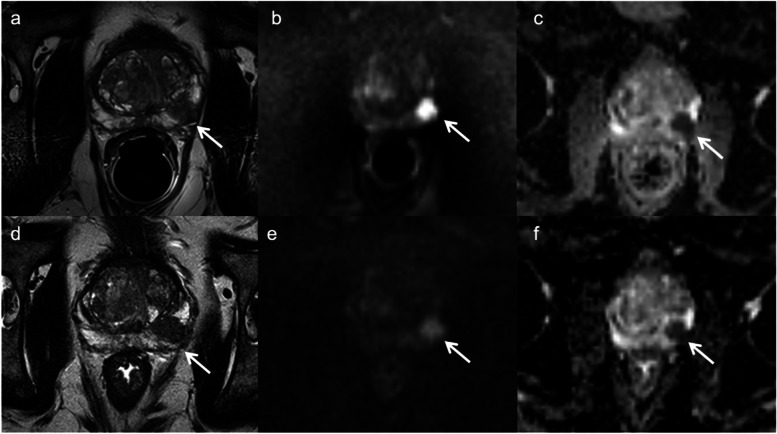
66-year old man (PSA of 9.03 ng/mL) who underwent MRI using a combined rigid two-channel phased-array endorectal coil and an external phased-array coil (ERC-PAC) (top row) and external PAC only acquisition (bottom row) with axial T2WI (a, d), DWI (b2000) (b, e) and ADC (c, f). A 15 mm PI-RADS 5 lesion (arrow) was identified in left mid-gland peripheral zone. Biopsy revealed Gleason 4 + 3 cancer in this location. Superior lesion detection and conspicuity are noted for ERC-PAC images. For this lesion, the estimated signal-to-noise (eSNR) for ERC-PAC vs. PAC alone were 11.6 vs 5.5  for T2WI and 36.3  vs 5.8 for b2000 DWI, respectively
